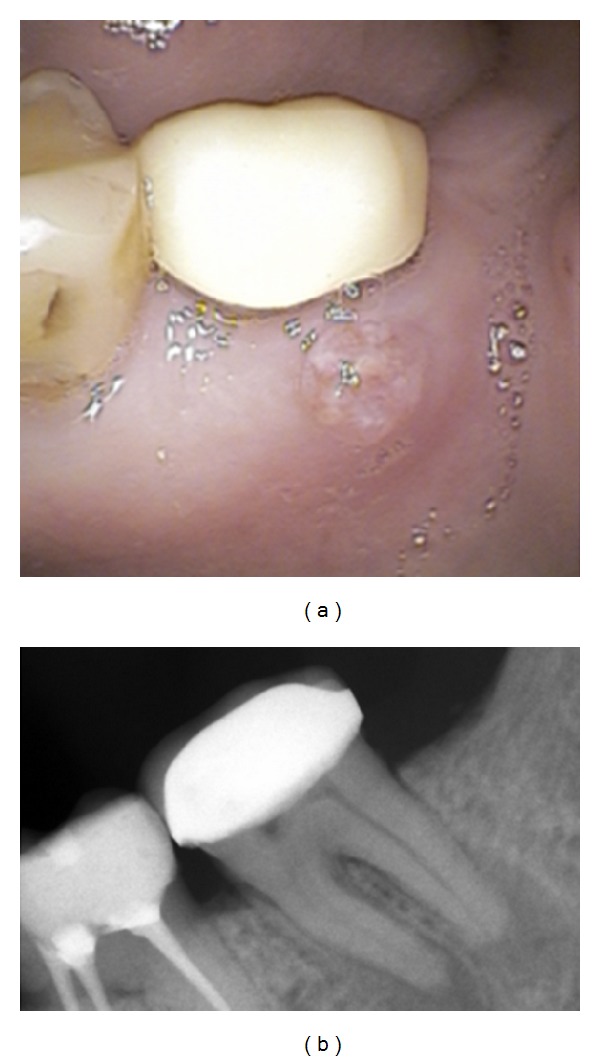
(a) Clinical image. Chronic suppurated apical periodontitis of tooth 37. Notice the fistula in the buccal area of this molar. (b) Radiological image. Notice the periapical radiolucent lesion in tooth 37.
